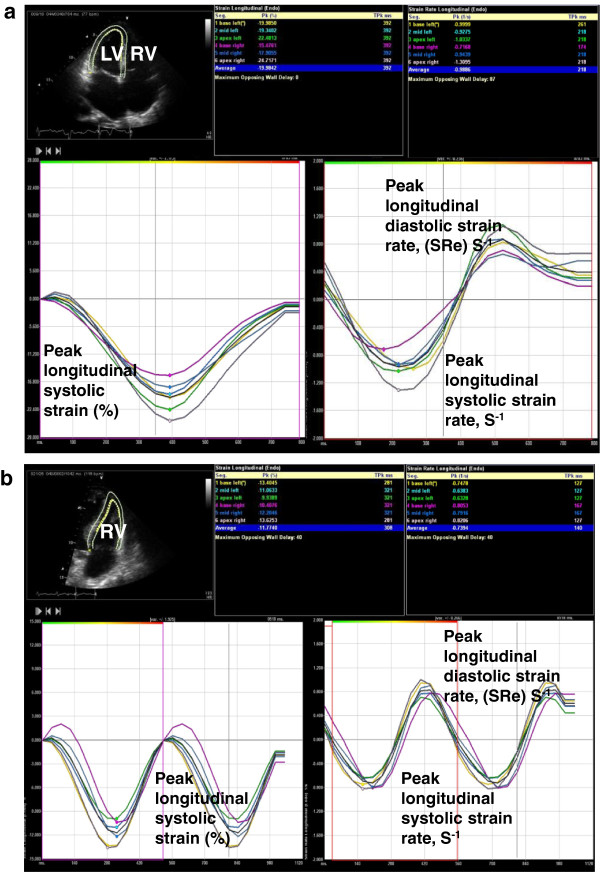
Longitudinal strain and strain rate curves. (a) Representative recording for apical four-chamber longitudinal strain and strain rate curves for a patient with normal left ventricle (LV) systolic function. Echo image displayed in Mayo format: left, LV; right, right ventricle (RV). Negative strain values indicate tissue contraction. Strain rate determined by change in strain over time. (b) Representative recording for apical four-chamber RV longitudinal strain and strain rate curves for a patient with abnormal RV systolic function. Echo image displayed in Mayo format: left, LV; right, RV. RV free wall longitudinal strain determined by the average of base, mid and apical free wall segments.
